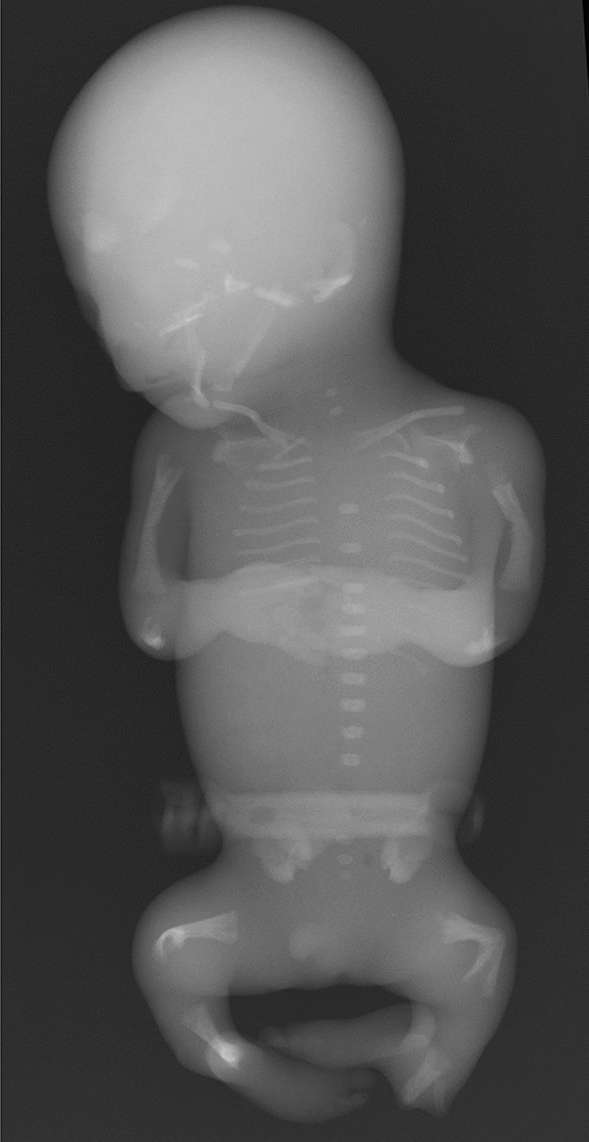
A stillborn with hypophosphatasia. Radiograph shows near-absent ossification of the skull (yet the frontal bones are preserved), short and thin ribs, small scapulae, absent ossification of the pubic and ischial bones, and sharp angulation of the long bones with metaphyseal defects extending into the diaphysis. Vertebral ossification is unequal. Some vertebral bodies are completely unossified, while others are well-ossified. The neural arches are thoroughly missing
